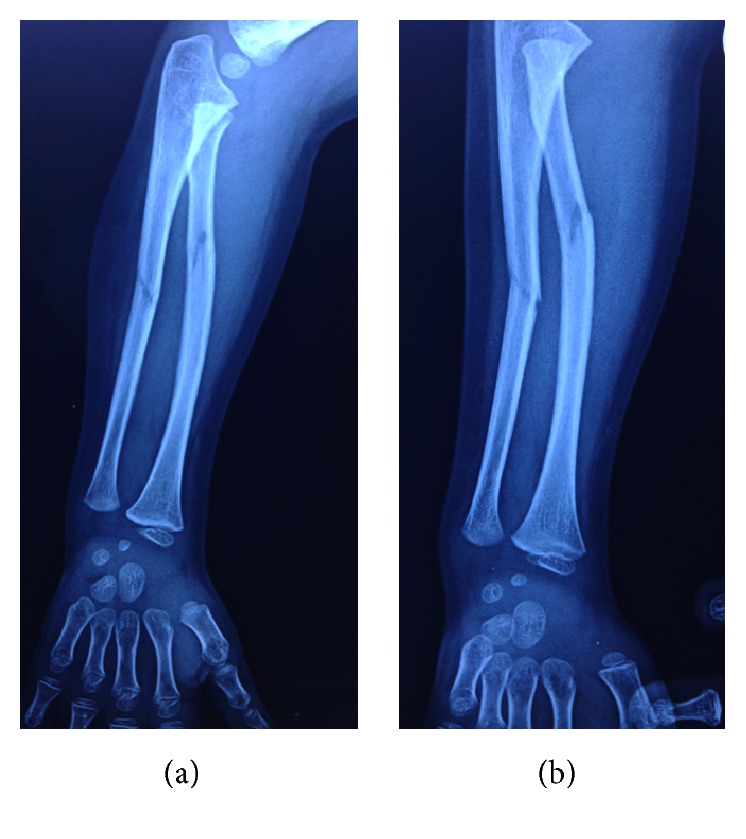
Oblique forearm radiographs taken in outside clinic revealed both bone forearm fractures and no anterior displacement of radial head.
